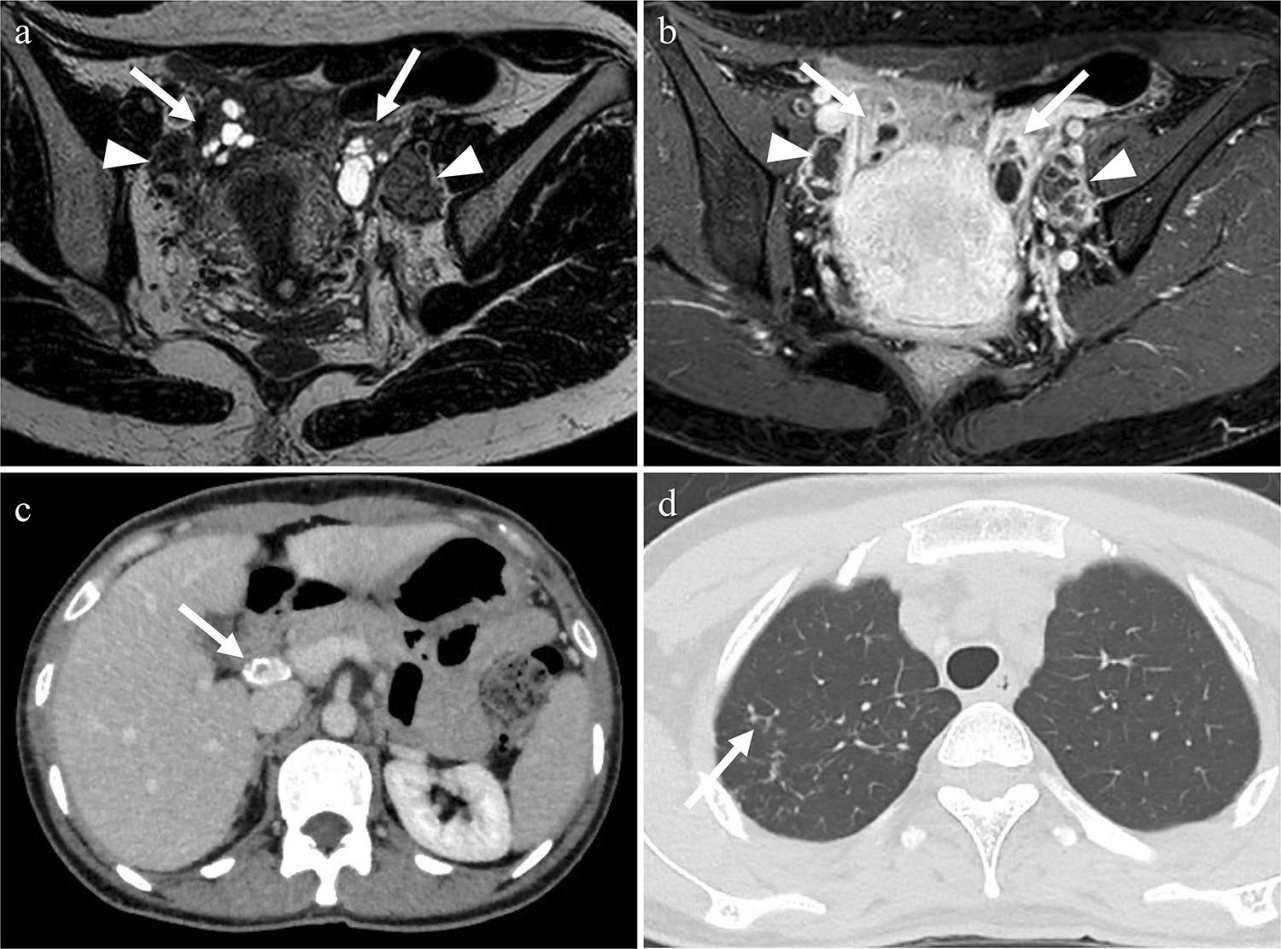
Woman in her 30 s with tuberculous tubo-ovarian abscesses. a Multiple cysts with septal thickening in both adnexa (arrows) and bilateral obturator lymph node swelling (arrowheads) are seen on the T2-weighted image. b The septa are strongly enhanced on fat-saturated contrast-enhanced T1-weighted image (arrows), indicating abscesses. Necrotic pelvic lymph nodes with a central contrast defect (arrowheads) are also demonstrated and tuberculosis is suspected. c, d Calcified hilar lymph node of the liver (arrow in c) and centrilobular nodules (tree-in-bud appearance) (arrow in d) in the right upper lobe of the lung on CT are also supportive of tuberculosis
